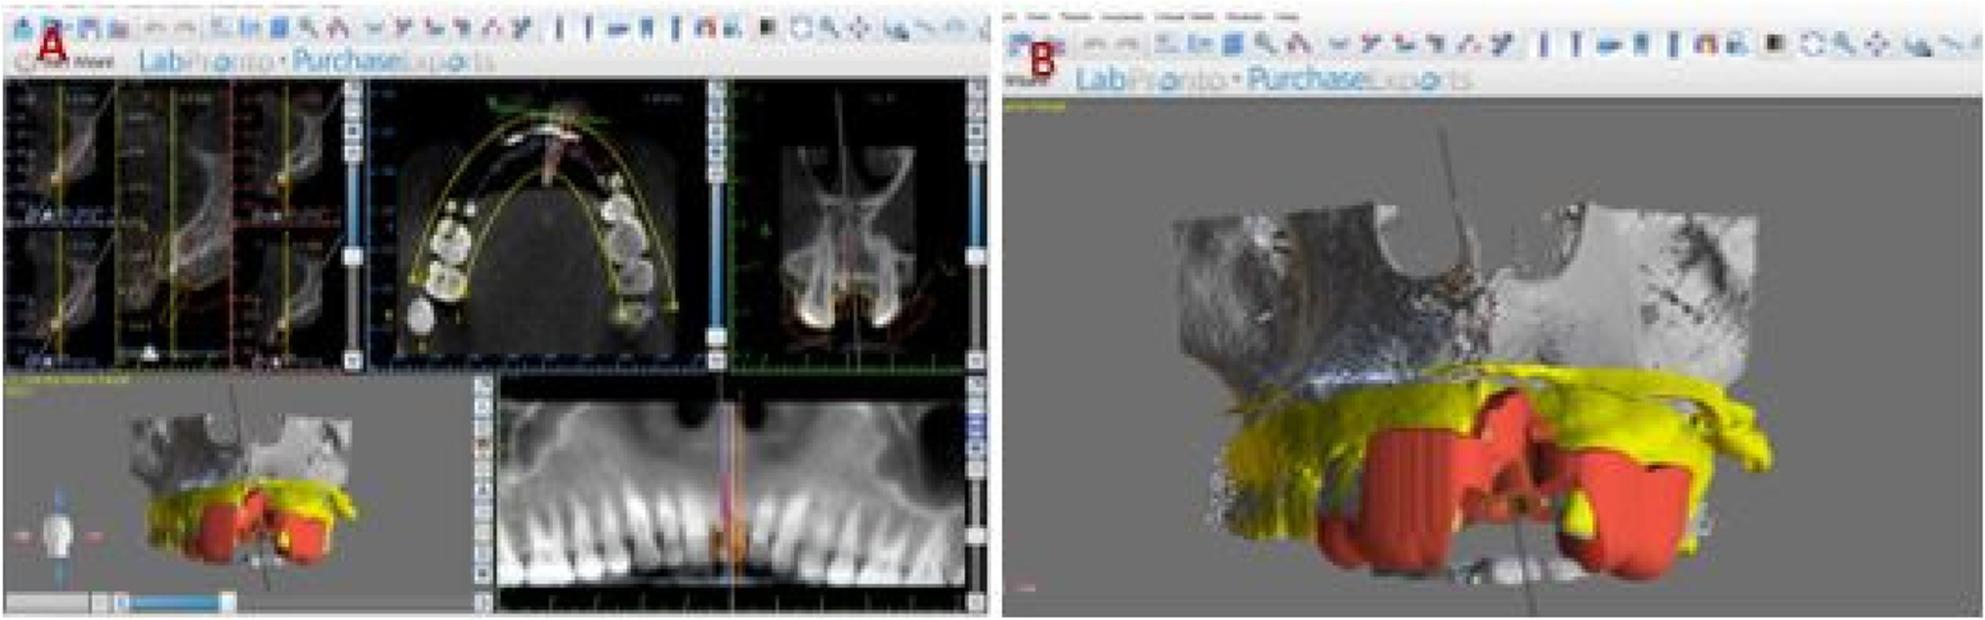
A Screenshot from Blue Sky Plan software demonstrating importation of DICOM files for virtual implant planning. Osteotomy positioning was planned based on the proposed implant site, considering the mesiodistal space, occlusal plane, and inter-occlusal clearance. B STL file of the diagnostic cast superimposed on the CBCT-derived bone model for guided surgical planning.
